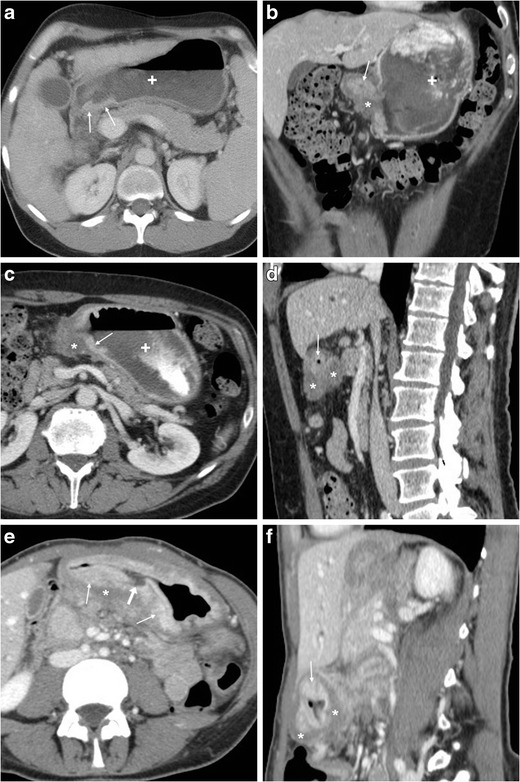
Differentiation of gastric tumours from PUD. In a 45-year-old HIV-positive male with wasting syndrome, CT (a) with oral water (+) showed localized, enhancing mural thickening at distal gastric antrum (thin arrows). In a 67-year-old male, CT (b…d) revealed distended gastric fundus (+) with residual contrast from previous fluoroscopic study, contracted antrum with mucosal enhancement (thin arrows) and hypoattenuating mural thickening (*); multiplanar image review revealed shouldering at transition between abnormal wall and upstream gastric body (in c) and eccentric, nodular-shaped thickening (in d) with subtle infiltration of perigastric fat, consistent with endoscopic diagnosis of stomach cancer. In a 44-year-old female, diffuse signet-ring gastric carcinoma (linitis plastica) showed CT (e, f) appearance of asymmetrically thickened, hypoattenuating wall (*) with irregular, enhancing mucosal thickening (thin arrows) and a focal posterior ulceration (arrow in e)
